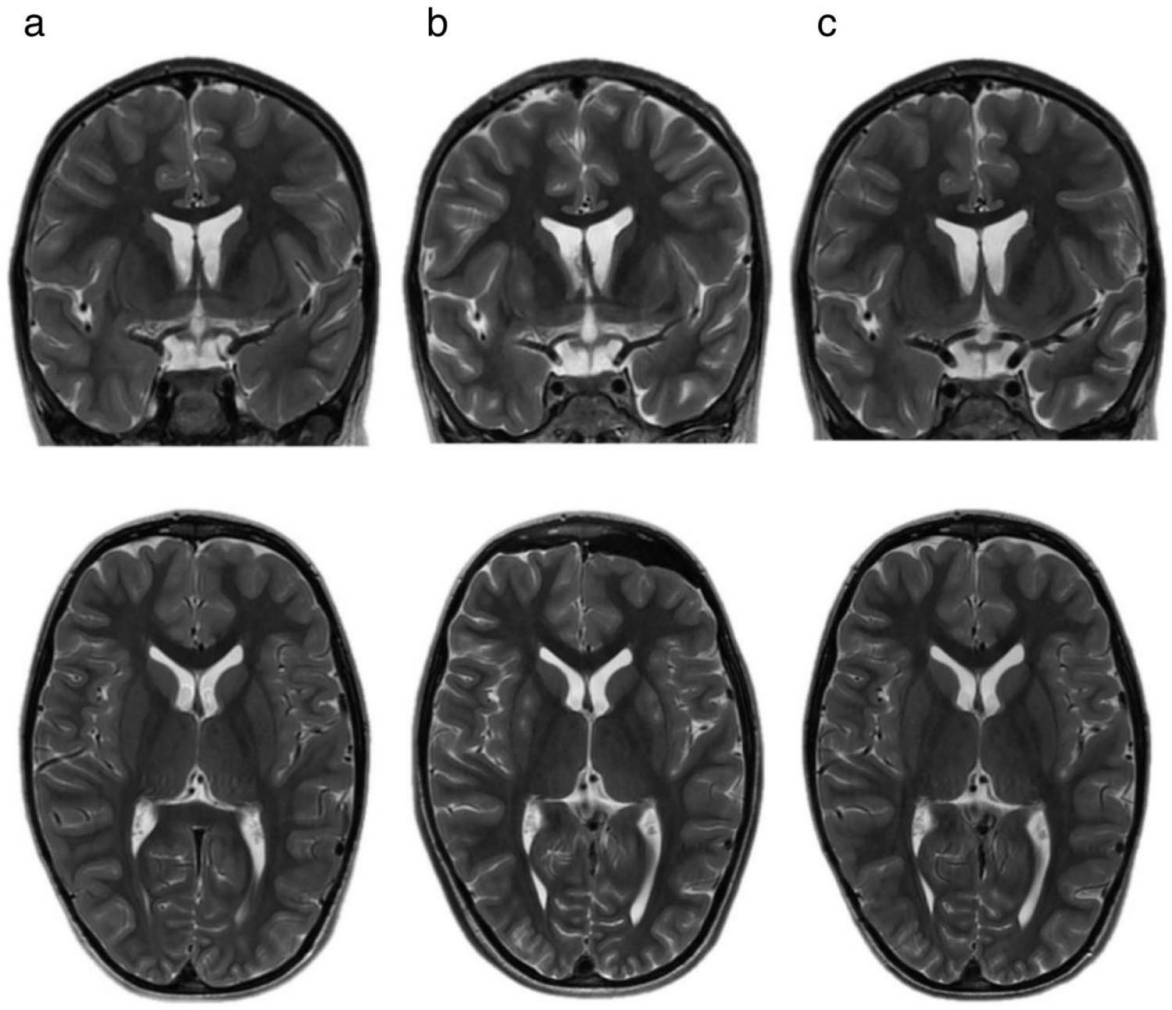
MRI scans of P1: A patient with preserved brain volume. a Preoperative T2-weighted images in frontal and axial views. b Postoperative T2-weighted images showing post-puncture trajectories. c T2-weighted images on postoperative day 30, demonstrating the absence of abnormalities or post-puncture tracts
